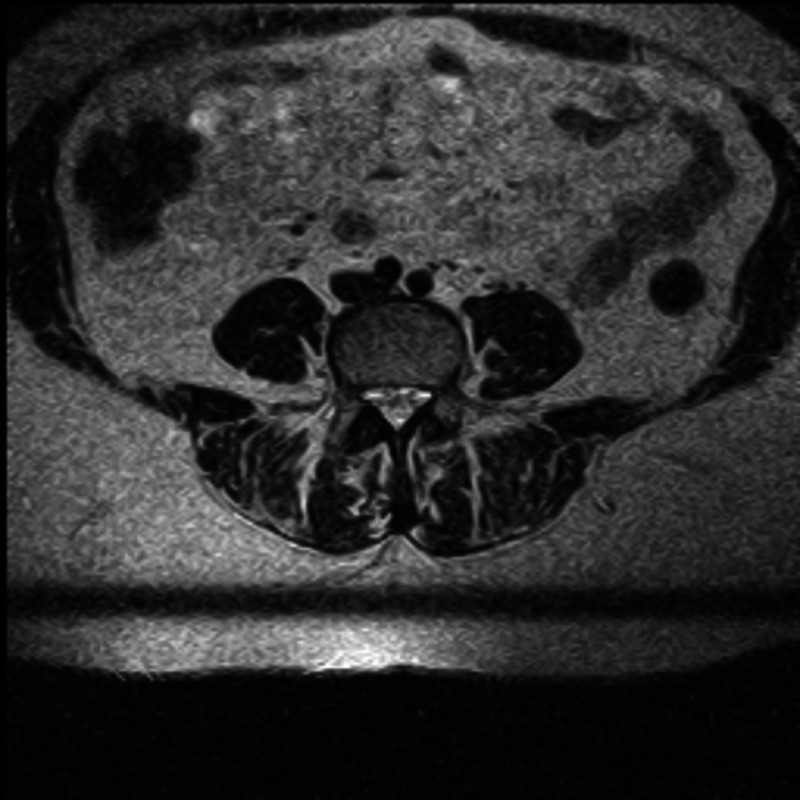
Axial T2 weighted MRI image with positive nerve root sedimentation sign.
